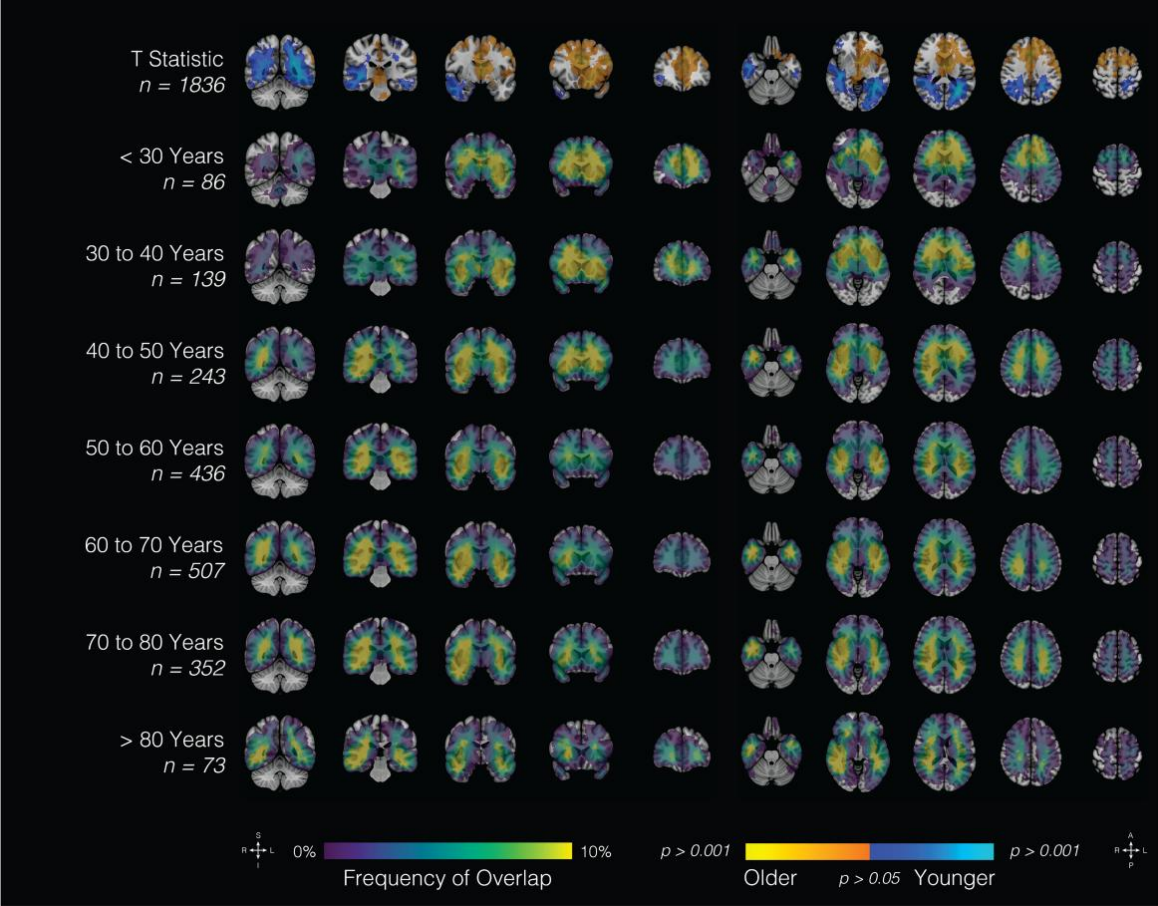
Age at MRI and location. Probabilistic maps showing spatial distribution of gliomas by age (*t*-statistic, continuous variable). Results are overlaid on the coronal (left half of the image) and axial (right half of the image) T1-weighted MNI brain. Scales indicate *t*-values (first row) and frequency of tumour overlap in percentages (bottom seven rows). MNI, Montreal Neurological Institute.
